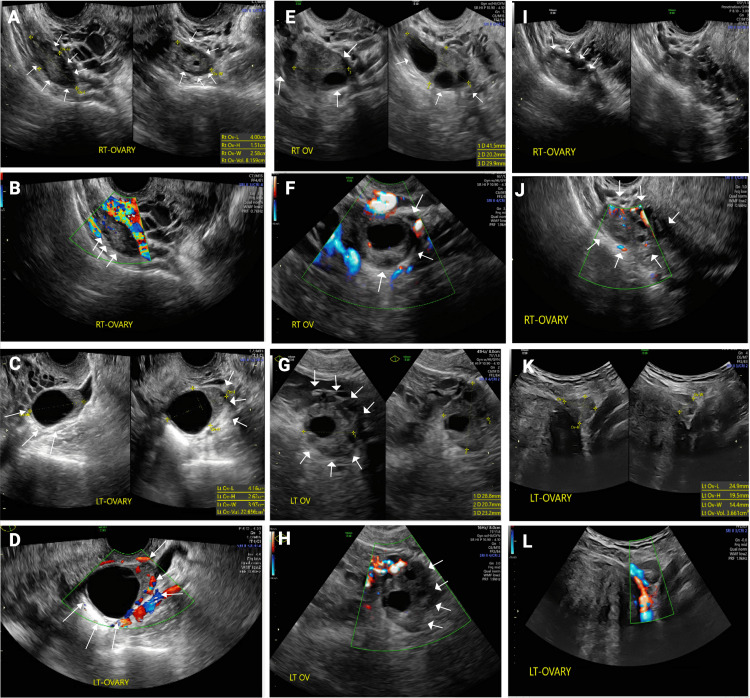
Serial annual grayscale and Doppler pelvic ultrasound scans spanning five years before the final follow-up visit A-D represent images from five years prior, obtained during a previous pregnancy, 18 months before starting osimertinib. E-H are from three years prior, and I-L are from one year prior to the final follow-up visit. A-D images were obtained during the first trimester of a previous pregnancy for comparison. A, B, E, F, I, and J represent the Rt. ovary, while C, D, G, H, K, and L represent the Lt. ovary. Ovarian images demonstrate multiple antral and growing follicles at various stages of development, indicated by well-circumscribed black circles (ovaries demarcated by white arrows). A&B, E&F, and I&J demonstrate the right ovary containing multiple antral follicles, indicating a good ovarian reserve. C&D demonstrate the left ovary containing a simple ovarian cyst, and G&H show a growing follicle in the Lt. ovary; hence, antral follicles were not visualized in these images. K & L are transabdominal ultrasounds, with L showing increased Doppler flow indicative of increased pelvic vascularity around the left ovary. Scale bars represent approximately 1 mm.
